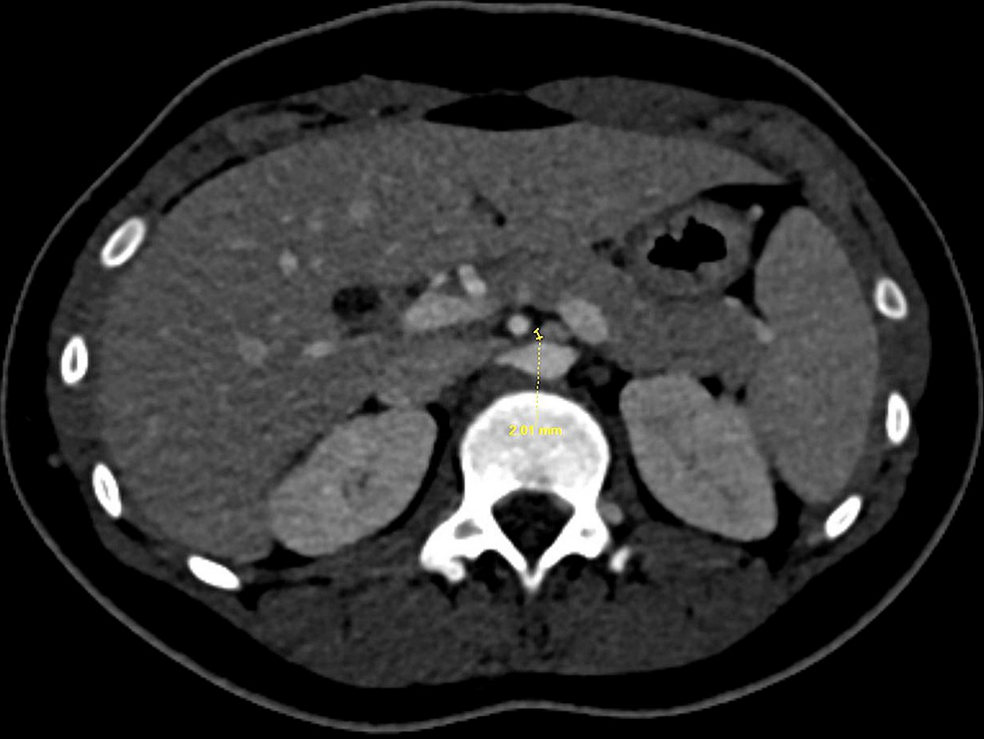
Axial CECT shows a 2-mm AP diameter of the LRV as it crosses between the aorta and SMA. CECT - Contrast-enhanced computed tomography; AP - anteroposterior; LRV - left renal vein; SMA - superior mesenteric artery
